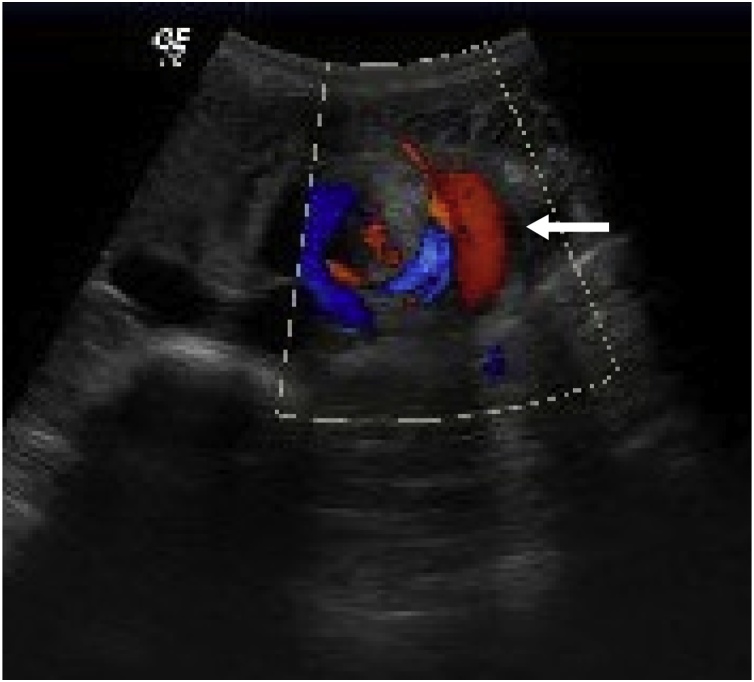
Abdominal ultrasound with whirlpool sign (white arrow) - hallmark of superior mesenteric vessels torsion.
